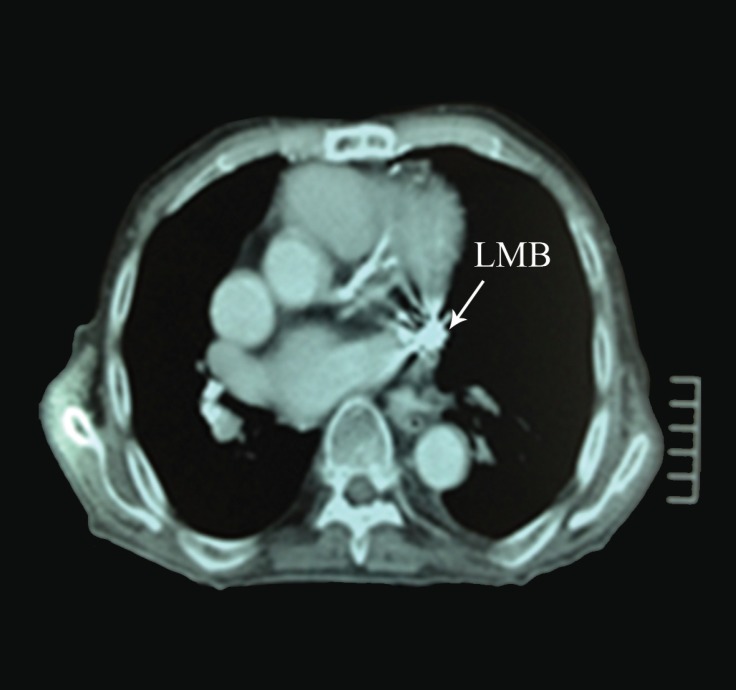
Computed tomography, showing the epicardial patch electrode in contact with the LMB (left main bronchus)
